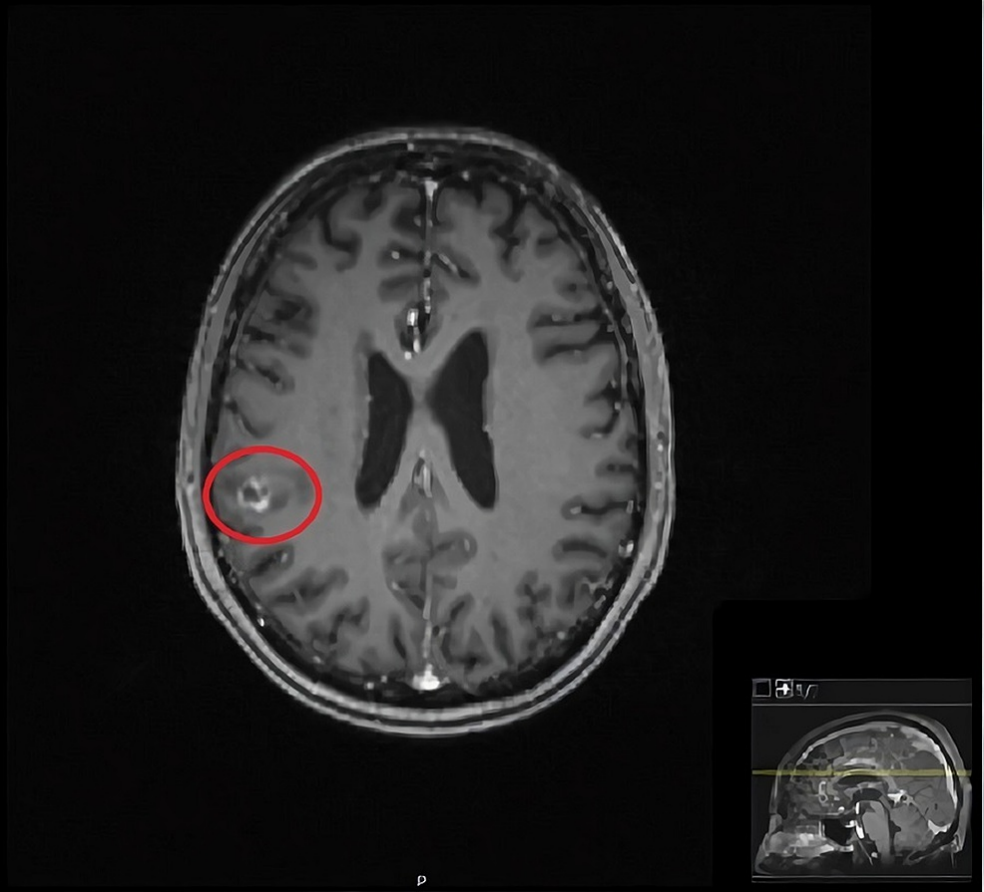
Cerebral MRI showing an intracranial tuberculous granuloma (red circle), with a ring of peripheral enhancement on T1-weighted contrast-enhanced image. MRI: magnetic resonance imaging
